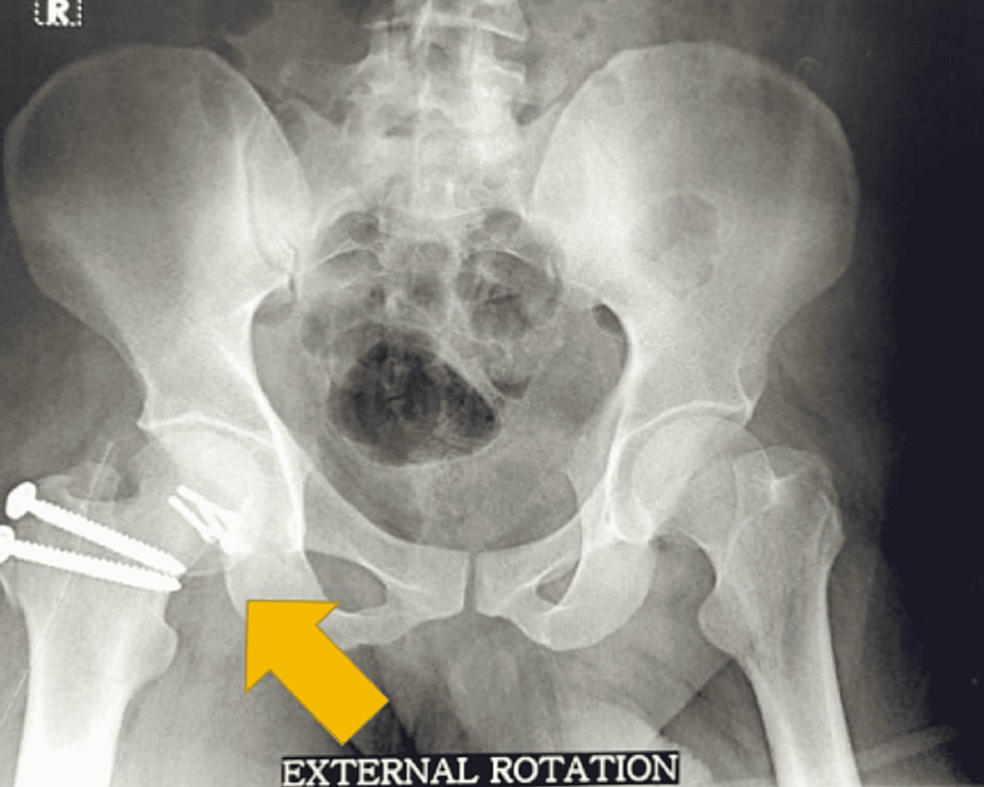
External rotation radiograph of pelvis anteroposterior view showing reduced Pipkin’s fracture at six months follow-up.
